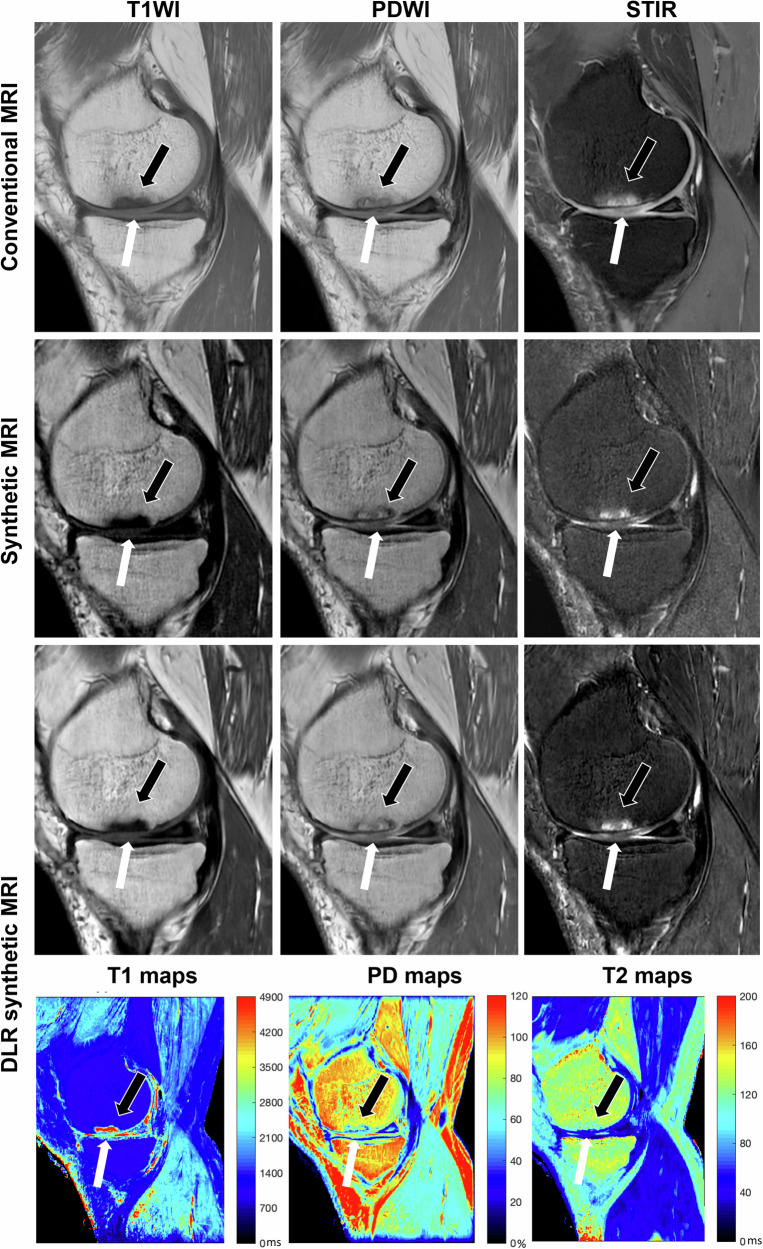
A representative image set of a 68-year-old man with right knee pain. Sagittal conventional and DLR synthetic knee MR images showed an inhomogeneous lesion both hypointensity and hyperintensity (white arrow) in the media femoral condyle accompanied by bone marrow edema (black arrow) while non-DLR SyMRI appeared vague lesion margins and more noises. STIR, short-tau inversion recovery; PD, proton density
